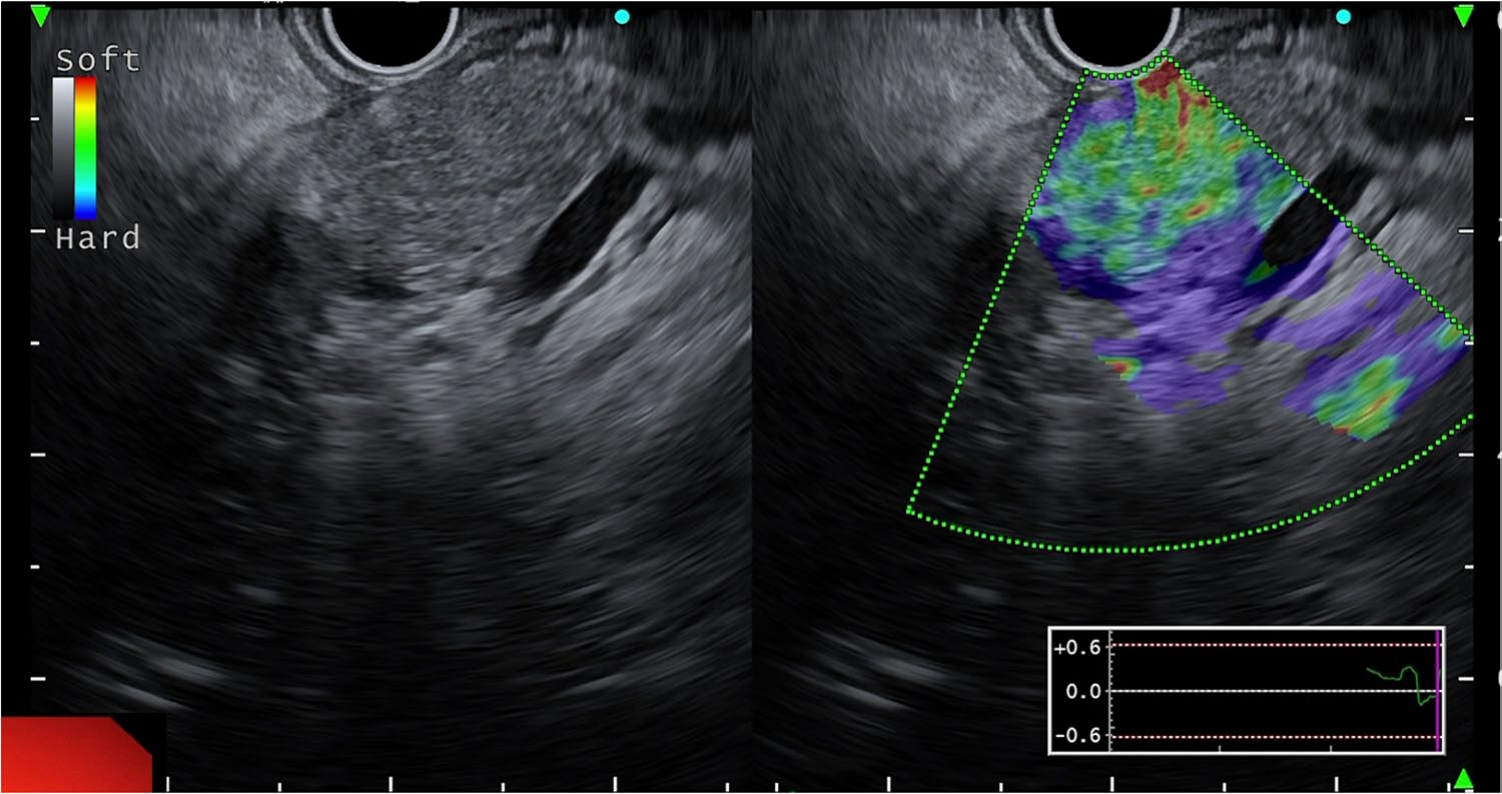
Endoscopic ultrasound showing heterogenous parenchymal echogenicity of the pancreas (left panel); On strain elastography the parenchyma appears diffusely firm (right panel)
